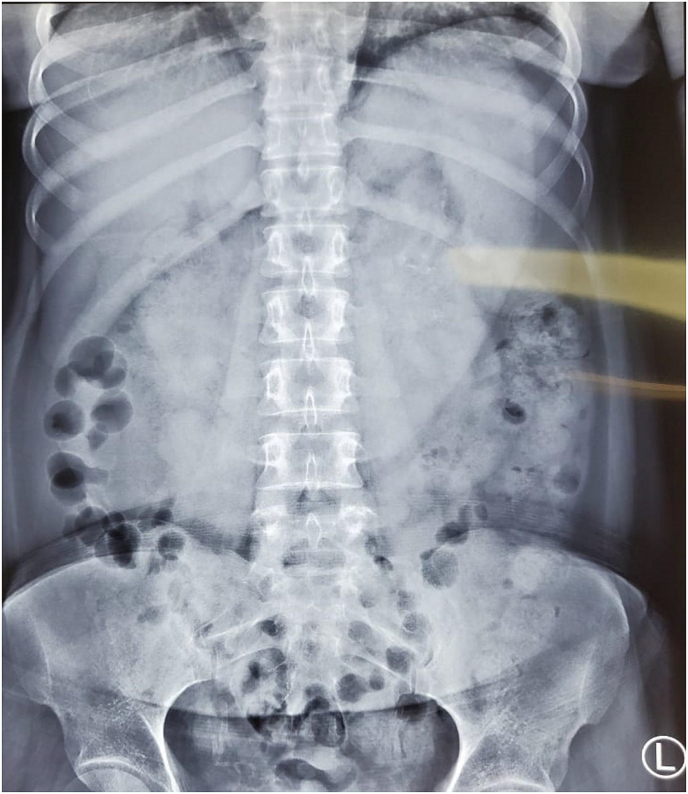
A plain abdominal radiograph showing a sizable soft tissue density with prominent stomach outline at left hypochondial region.
